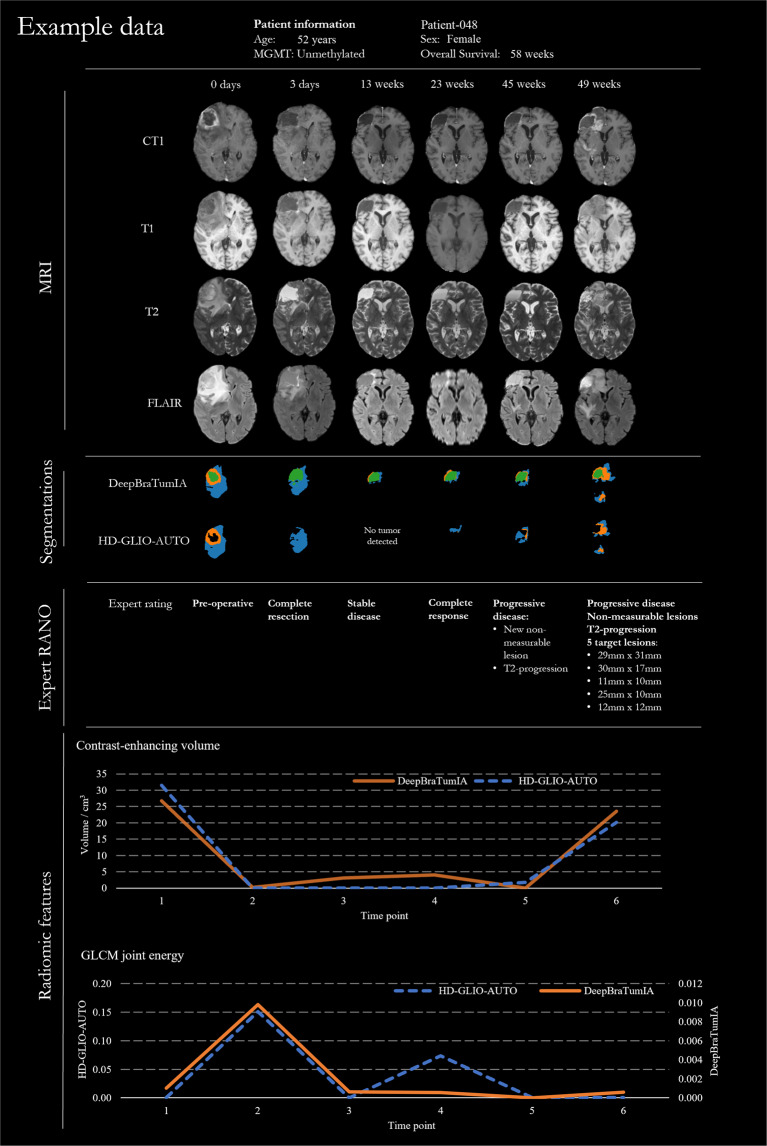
Example of longitudinal GBM data. CT1/T1: T1-weighted post-/pre-contrast, T2-weighted, and fluid-attenuated inversion recovery (FLAIR). For this patient, the whole contrast enhancement was completely resected. After a phase of complete response, progression occurred with a very fast growth rate.
